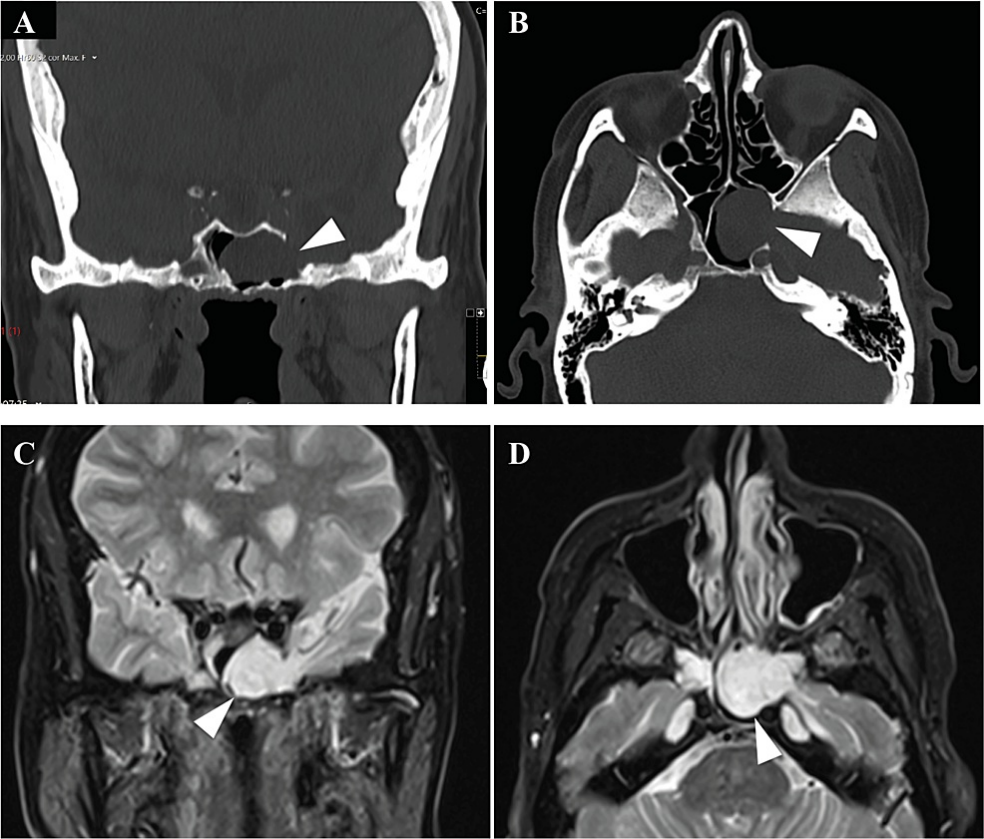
CT and MRI show dehiscence of the lateral left sphenoid wall with brain herniation. CT scans (A and B) show dehiscence of the lateral left sphenoid wall (arrowheads), establishing communication with the middle cranial fossa. MRI (C and D) shows bone discontinuity in the left lateral wall of the sphenoid, crossed by tissue with signal emission characteristics similar to brain parenchyma and CSF to the left chamber of the sphenoid sinus (arrowheads).
